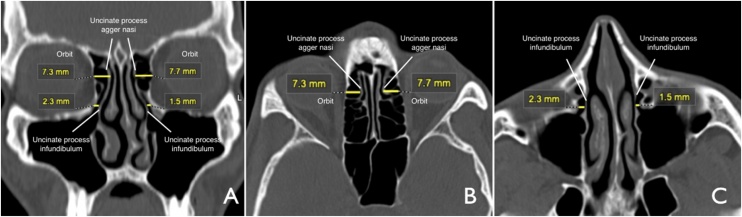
Computed tomography of the paranasal sinuses (bone window). Horizontal yellow lines measure the greatest distance from the uncinate process to the medial wall of the orbit at the level of the agger nasi and ethmoid infundibulum. A: coronal slice.
